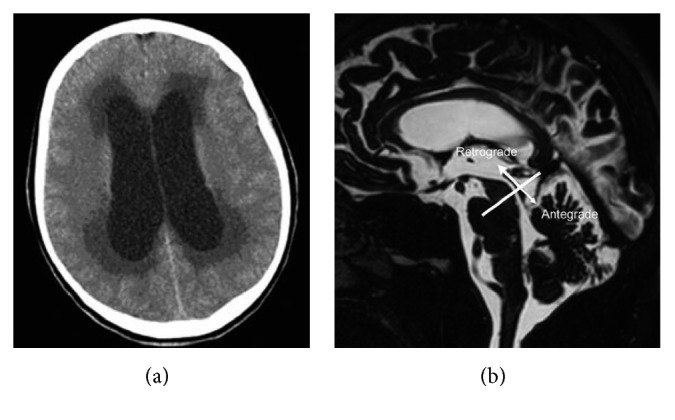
(a) Hydrocephaly image by CT scan (the image is obtained from Fitz and Harwood-Nash (1978)). (b) MRI obtained by steady-state technique (the image is obtained from Ringstad et al. [20]).
